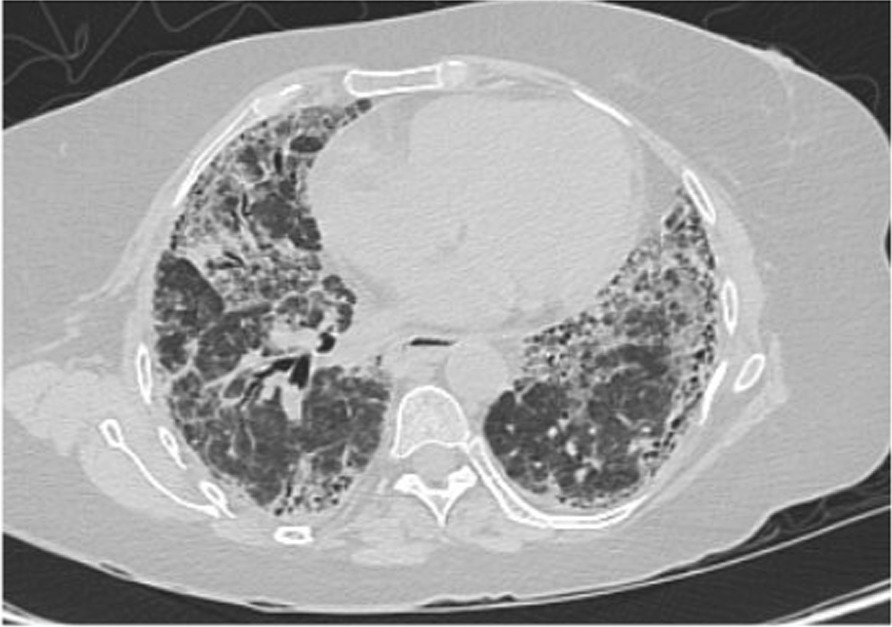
At day 30, high-resolution computed tomography showing interval improvement of bilateral ground-glass opacities with stable fibrotic changes
